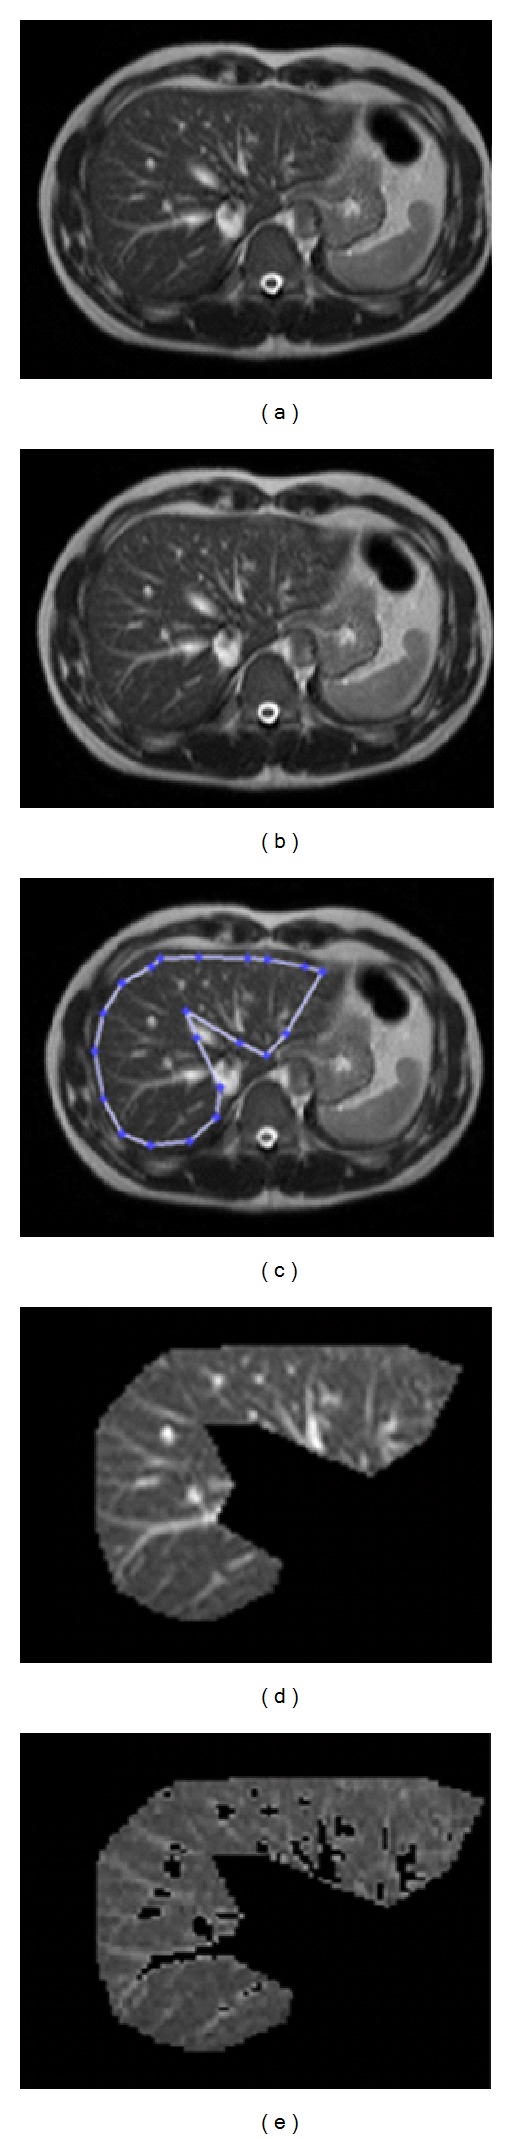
(a) MRI T2-weighted image (original scale), (b) MRI T2-weighted image on altered scale, (c) selected ROI of liver, (d) magnified ROI of liver, and (e) magnified ROI of liver after filtering out of artery and portal veins using Otsu's algorithm.
